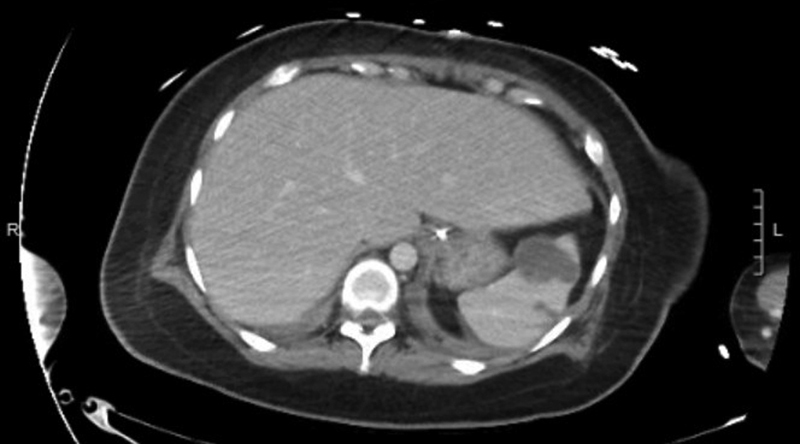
CT abdomen (axial view) with multiple infarcts in the spleen: largest lesion is 4.1 cm partially exophytic suspicious for hemorrhage into prior infarct. CT, computed tomography.
